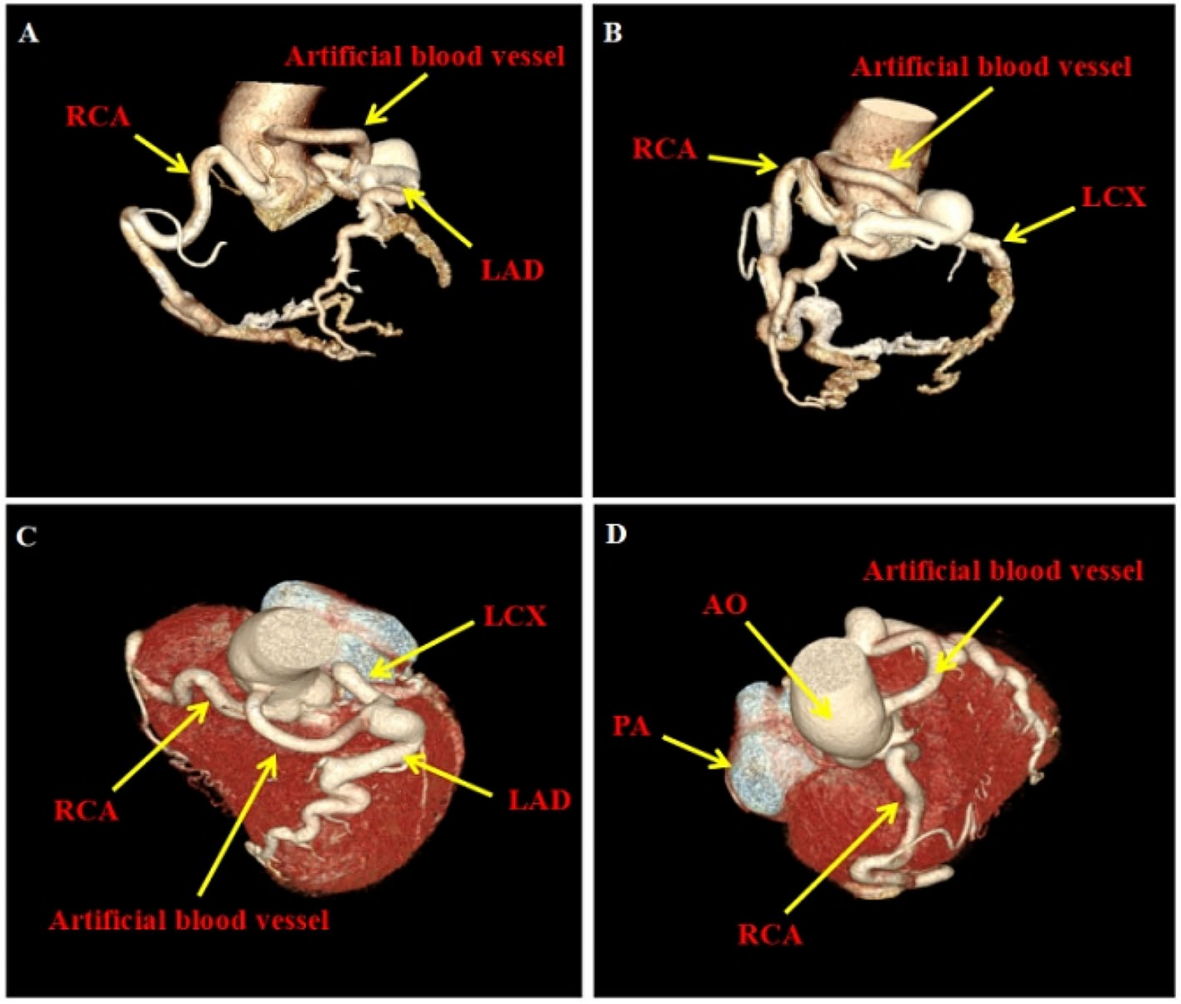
Postoperative coronary computed tomographic angiography. A-D The 6 mm GORE-TEX artificial vessel was connected to the left coronary artery and the aortic root for coronary branch dilation, and the coronary artery presented a right dominant type. AO: aorta, PA: pulmonary artery, LAD: left anterior descending branch, LCX: left circumflex branch, RCA: right coronary artery, Artificial blood vessel: artificial blood vessel
